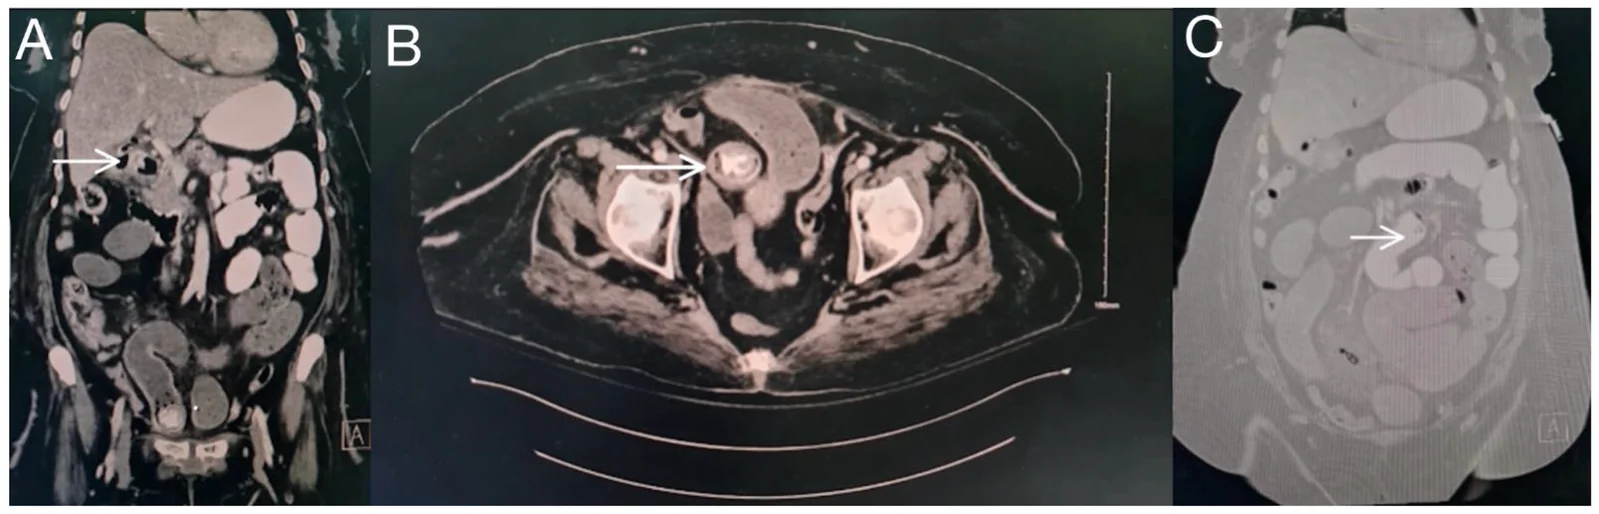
CT abdomen and pelvis: (A) coronal view showing the previously noted sizable gallstone not visualized in the gallbladder, with the gallbladder appearing contracted. Positive oral contrast is seen filling the gallbladder and the common bile duct (CBD); findings were suggestive of a bilioenteric fistula, most likely cholecystoduodenal, although the fistula was not surgically explored (arrow). Minimal pneumobilia is also noted. (B) Axial view showing the previously noted sizable gallstone (3.2 × 2.6 cm) now located in the distal ileum (arrow). (C) Coronal view demonstrating foci of extra-luminal air (arrow), suggestive of small bowel wall pressure necrosis or ischemia.
